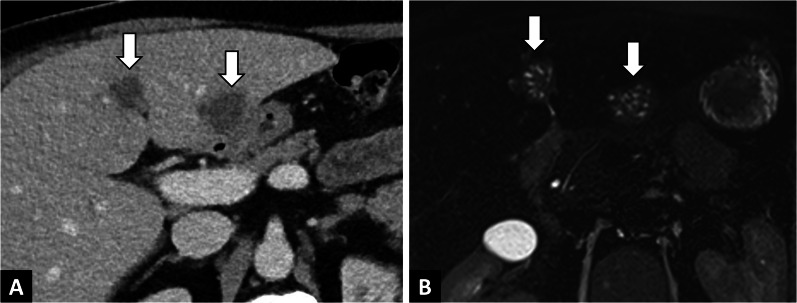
Atypical CT findings of alveolar echinococcosis in a 65-year-old woman with a recent diagnosis of lymphoma. A CT image in the axial plane showing two aspecific hypodense liver lesions of the left liver (arrows). B T2-weighted fat-suppressed MR image in the axial plane showing typical microcysts within hypointense T2 lesions (arrows; Kodama 2)
